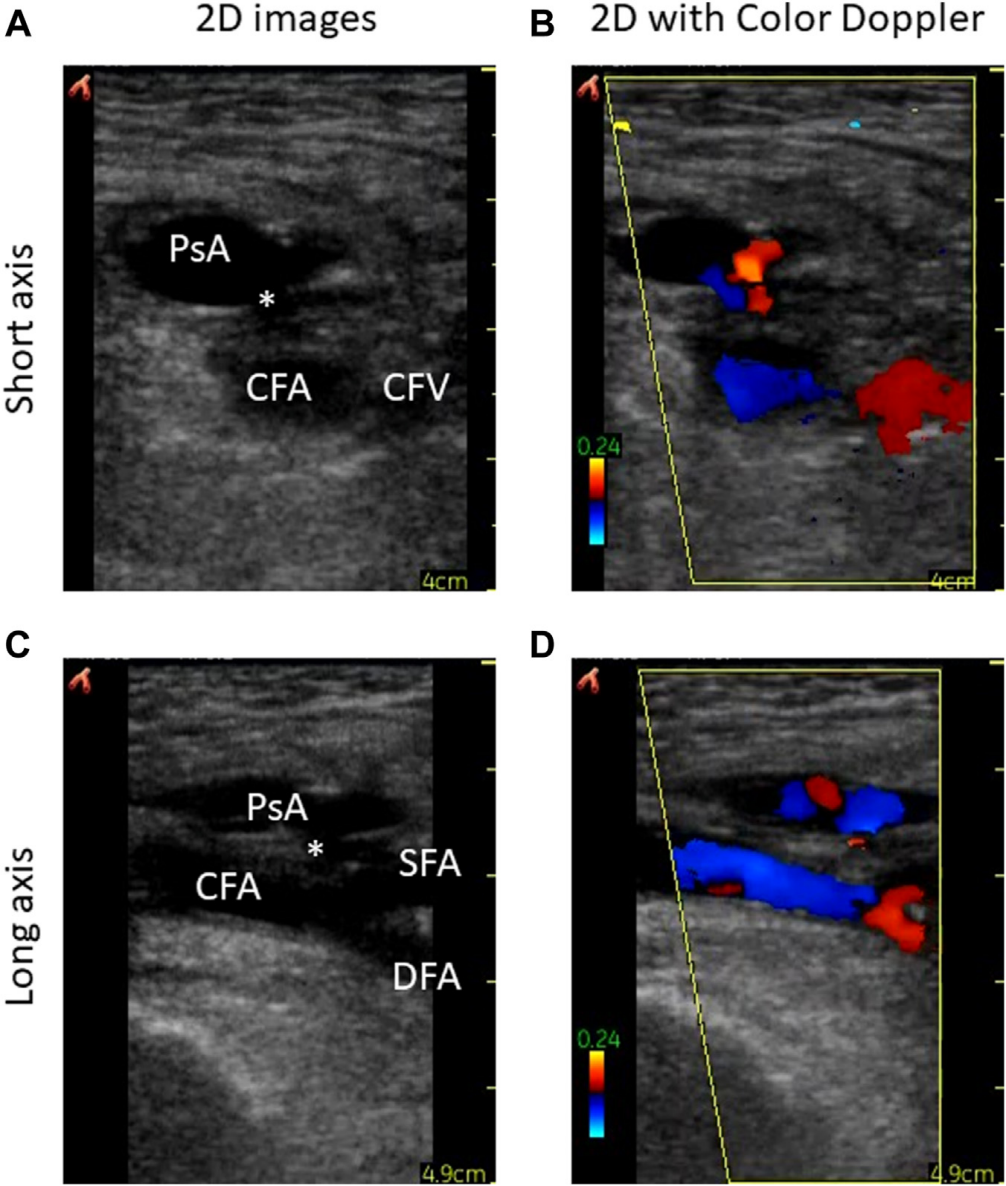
Pseudoaneurysm (PsA) identified on point-of-care ultrasound. PsA as visualized on point-of-care ultrasound with the neck of the PsA (∗) just proximal to the femoral artery bifurcation in the (A) short axis without color, (B) short axis with color Doppler, (C) long axis without color, and (D) long axis with color Doppler. CFA, common femoral artery; DFA, deep femoral artery; SFA, superficial femoral artery.
